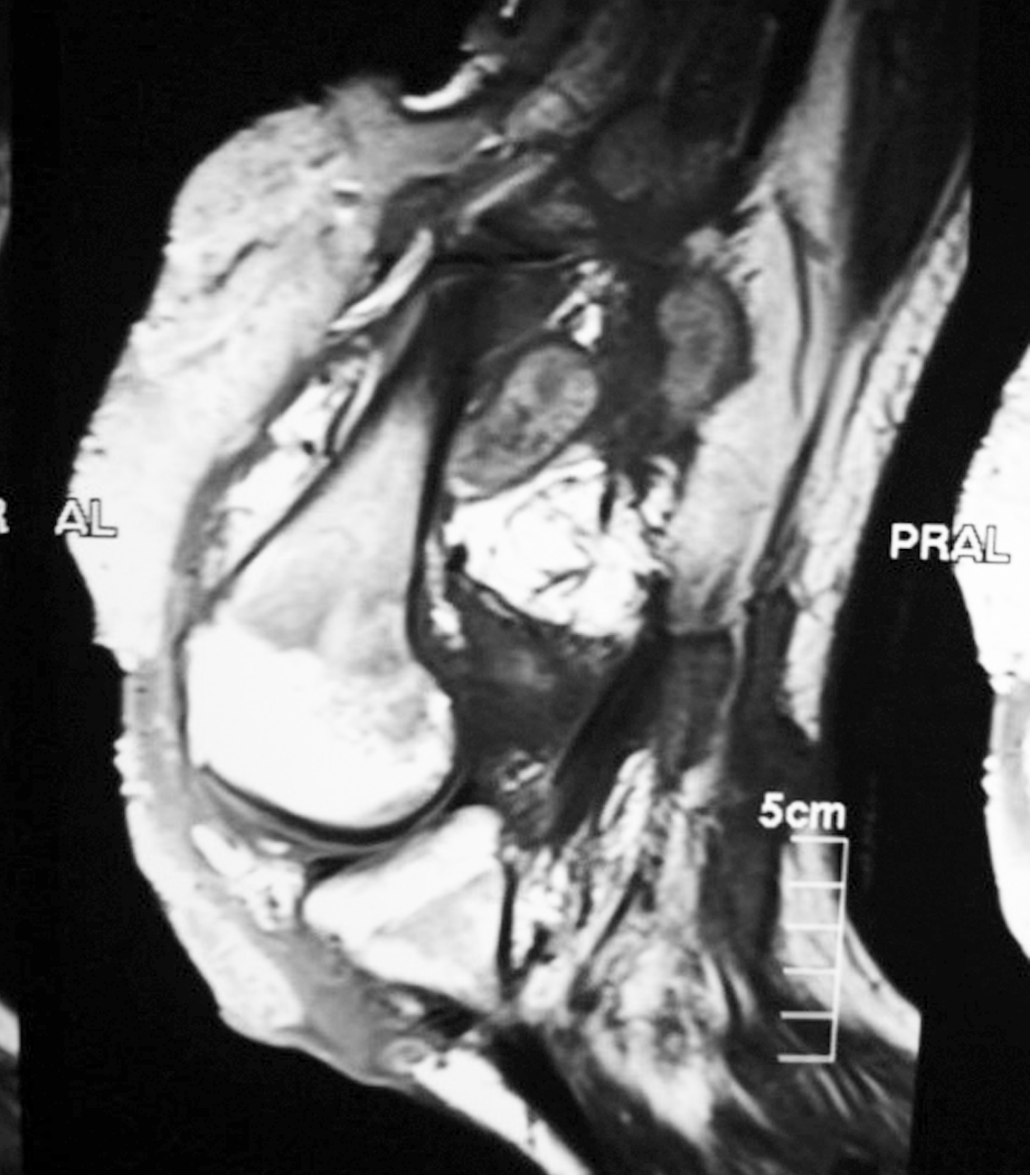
The knee region MRI showing extensive enhancing solid mass with nodule components, infiltrating the muscular components of anterior and posterior lower thigh with intra-articular extension and bony cortical defect at lateral femoral condyle.
